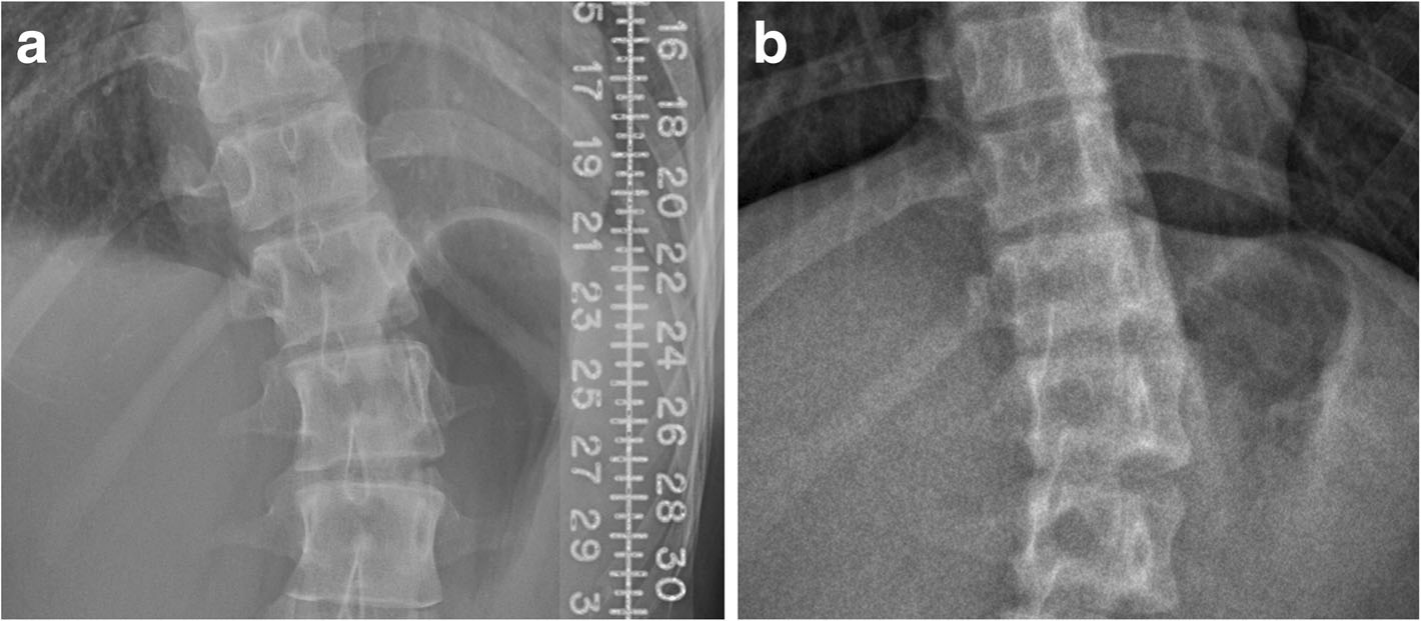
Image comparison between a standard digital radiography and b micro-dose EOS
